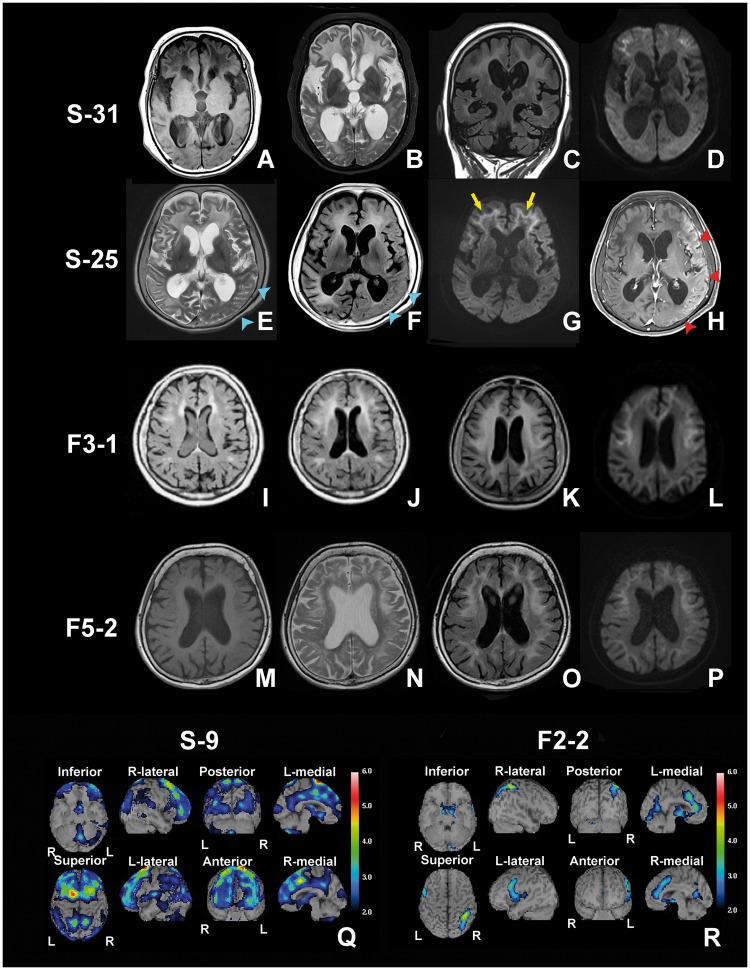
The results of NIID head MRI and SPECT. Head MRI findings of Subject S-31 (sporadic NIID) (A–D), Subject S-25 (sporadic NIID) (E–H), Subject F3-1 (familial NIID) (I–L) and Subject F5-2 (familial NIID) (M–P). (A and M) T_1_-weighted image. (B, E and N) T_2_-weighted image. (C, F, I–K and O) FLAIR image. (D, G, L and P) DWI image. (H) Gadolinium-enhanced T_1_-weighted image. (A–D) Head MRI findings of Subject S-31 at age 72 years, almost akinetic mutism state. Prominent leukoencephalopathy, DWI high intensity signal in corticomedurally junction and ventricular distention were observed (D). (E–H) Head MRI findings at the presence of encephalitic symptom of Subject S-25. On T_2_ (E) and FLAIR (F) image, brain oedema is observed (blue arrowhead). On DWI, oedematous portion showed slightly high intensity but weaker than corticomedurally junction high intensity signal (yellow arrow) (G). Oedematous portion showed high intensity on gadolinium enhanced T_1_ image (red arrow head) (H). (I–L) Progress of leukoencephalopathy in familial NIID Subject F3-1 at age 53 (I), age 58 (J) and age 64 (K and L). High intensity area of FLAIR image extended remarkably. (M–P) Head MRI findings of familial NIID Subject F5-2 presented dementia dominantly. Findings are almost identical to sporadic case. (Q and R) The results of easy Z-score imaging system (eZIS) analysed data of ^99m^Tc-ECD SPECT. Subject S-9 showed severe regional cerebral blood flow decline and Subject F2-2 eZIS data showed light regional cerebral blood flow decline.
